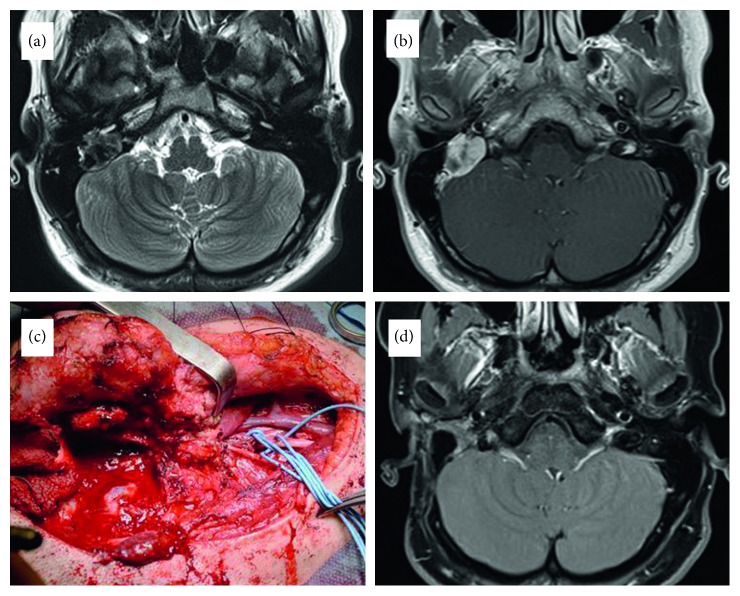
Pre- and postoperative MRI scans of Case 1. (a) Preoperative MRI T2 sequence showing the tumour measuring 25 × 20 mm at the jugular foramen with infralabyrinthine extension causing erosion of the posterior semicircular canal. Note clear distinction between the tumour and cerebellum posteriorly. (b) Preoperative MRI T1 with gadolinium enhancement. (c) Intraoperative picture after extended mastoidectomy and neck dissection. The next step was anterior rerouting of the facial nerve, which gives required access to the jugular foramen. (d) Postoperative MRI T1 with gadolinium enhancement after 3 years of follow-up. Total tumour clearance and no evidences of recurrence could be confirmed.
